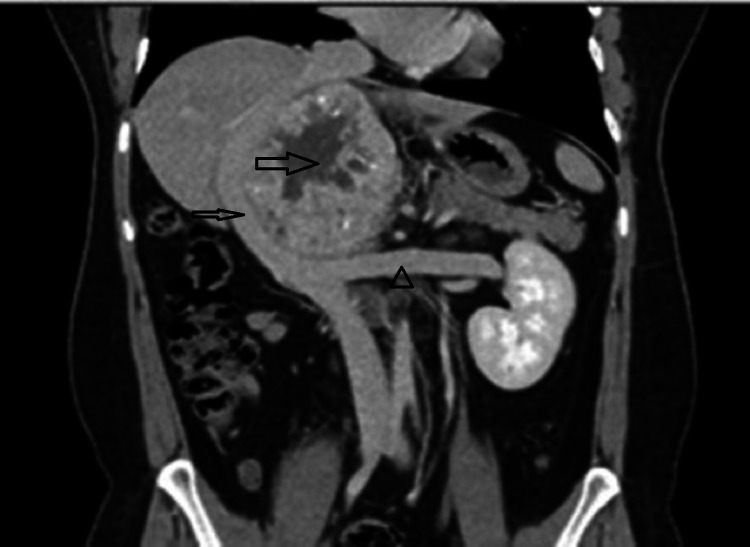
CT scan (coronal view) showing huge right retroperitoneal tumor with central necrosis (thick arrow) pushing the IVC (thin arrow) anteriorly to the right. The left renal vein (arrowhead) is being pushed inferiorly. The IVC and left renal vein are being stretched over the tumor without signs of an invasion. IVC: Inferior vena cava.
